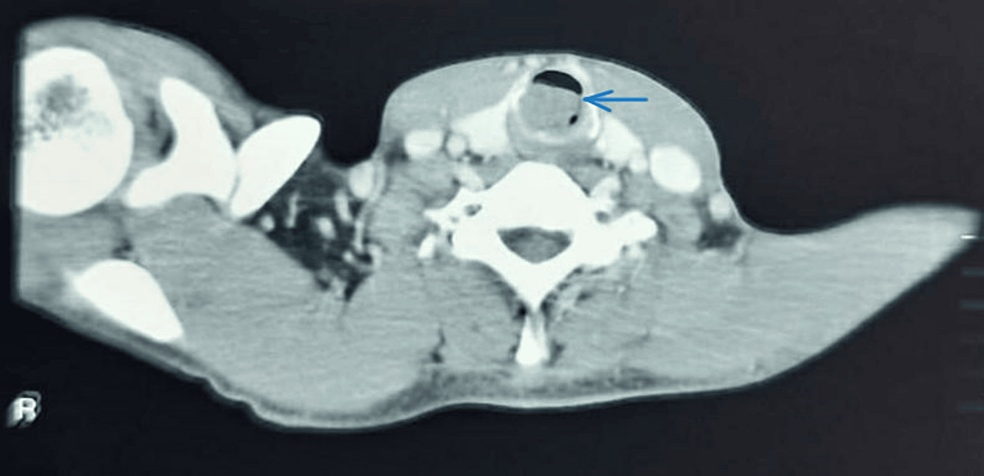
CECT revealing a round to oval well-defined soft tissue density mass lesion arising from the posterolateral aspect of the trachea in the subglottis region with posterior extension CECT, contrast-enhanced computed tomography
